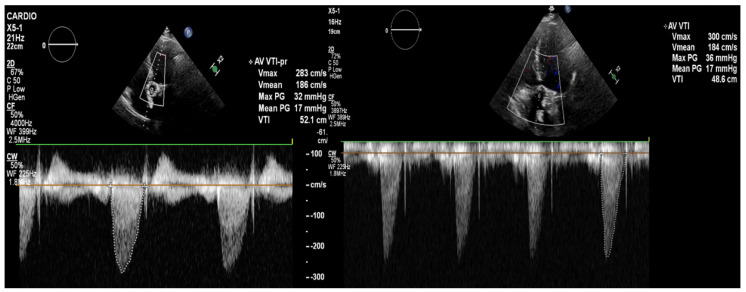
The transthoracic echocardiography showed a reduction in the gradients through the aortic valve prosthesis with parameters similar to that after the TAVI (on left: post-TAVI; on right: post-LMWH treatment). According to the 2021 ESC/EACTS Guidelines, in patients after TAVI, lifelong SAPT is recommended in the lack of indications for oral anticoagulants (OACs) [1]. However, despite similar recommendations for aspirin, the 2020 ACC/AHA Guidelines suggest that in patients with a low bleeding risk, it is worth acknowledging the antithrombotic prophylaxis with DAPT or VKA [2]. The direct-acting oral anticoagulants (DOACs) were not found to be superior to the administering antiplatelet or VKA, and in patients without indications for OAC, were linked to a higher incidence of all-cause mortality [3,4,5]. The 2021 ESC/EACTS and 2020 ACC/AHA Guidelines for managing valvular heart disease indicate that anticoagulation using VKAs or UFH is a first-line therapy for bioprosthetic valve thrombosis. Such an approach is highly effective in the normalization of valve function in 85% of the patients [6].
